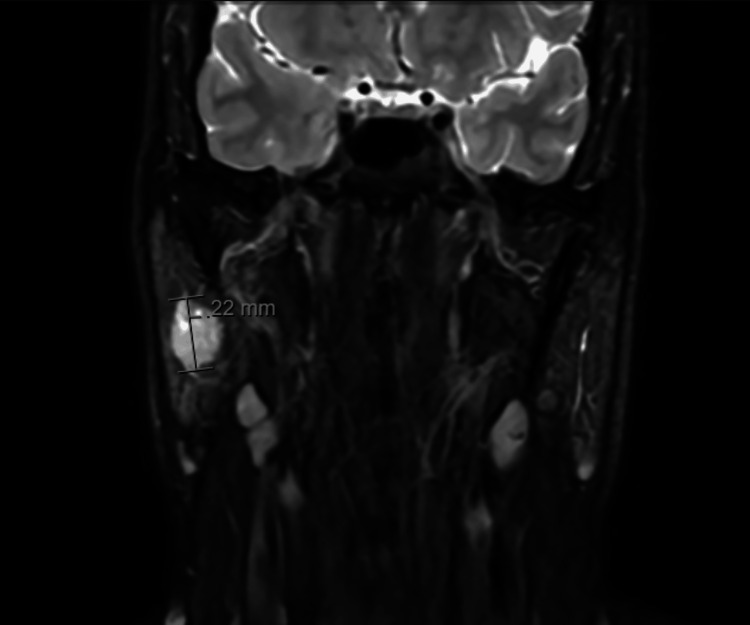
Coronal MRI neck showing parotid lesion
